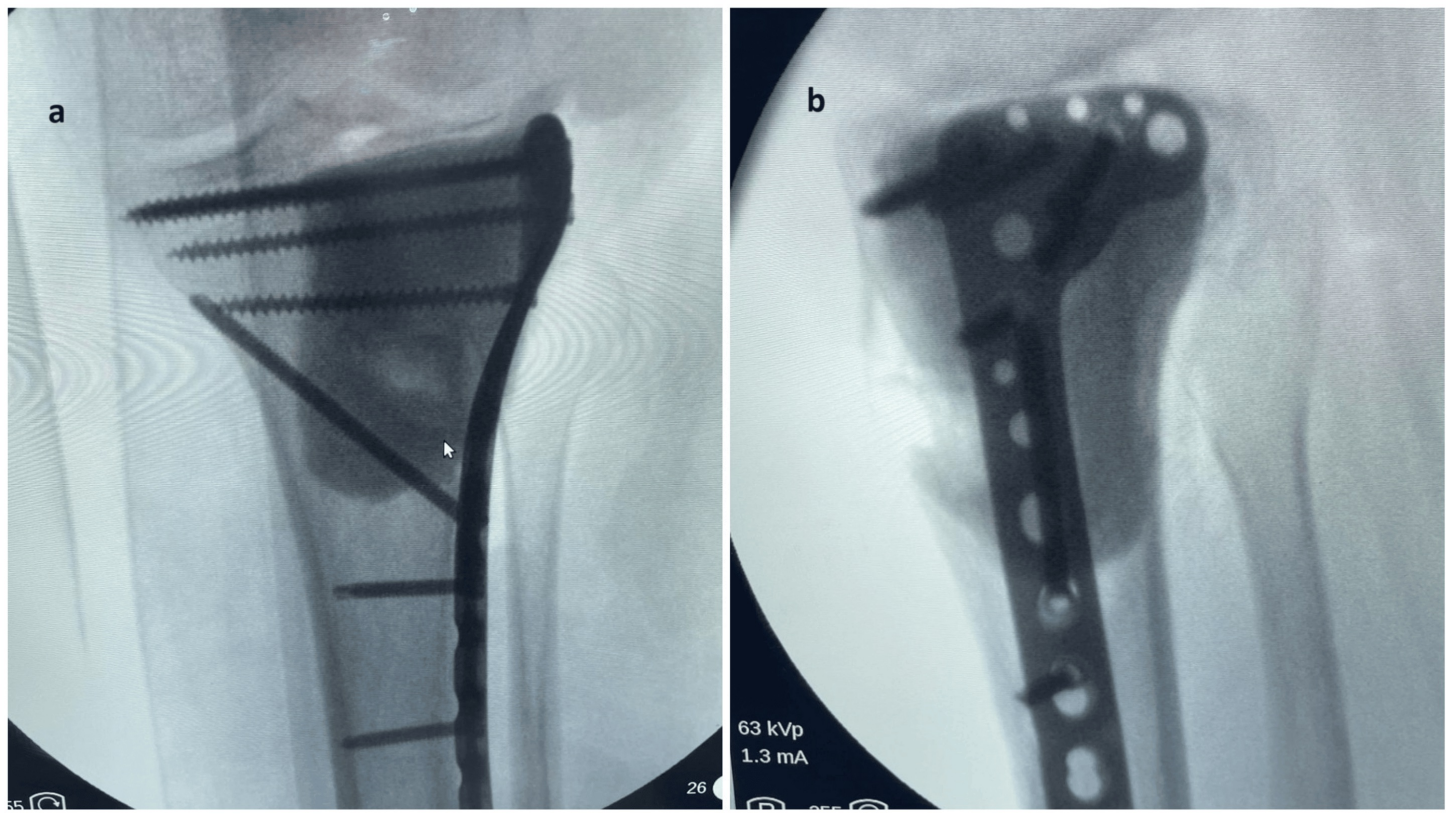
Intraoperative fluroscopy images Intraoperative radiographs after the completion of the procedure (a: anteroposterior view; b: lateral view)
